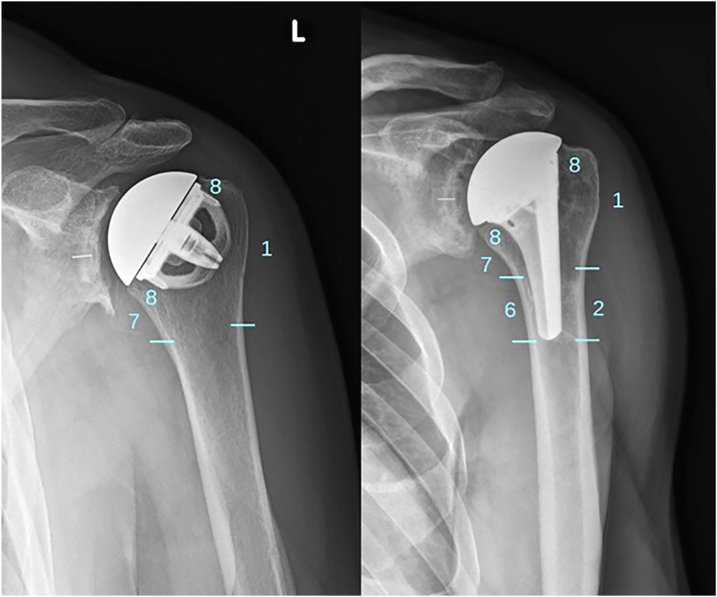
Radiographic zones of post-operative radiolucent lines. *Left*: stemless implants impact only the (1) greater tuberosity, (7) medial calcar, and (8) collar regions. *Right*: short-stem implants also reach the (2) lateral metadiaphyseal and (6) medial metadiaphyseal zones.
